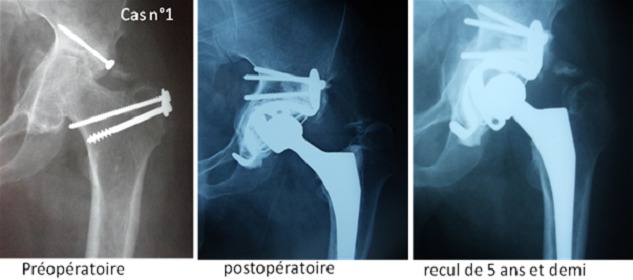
Radiographies de la hanche chez l'un de nos patients montrant la bonne intégration de la greffe osseuse
